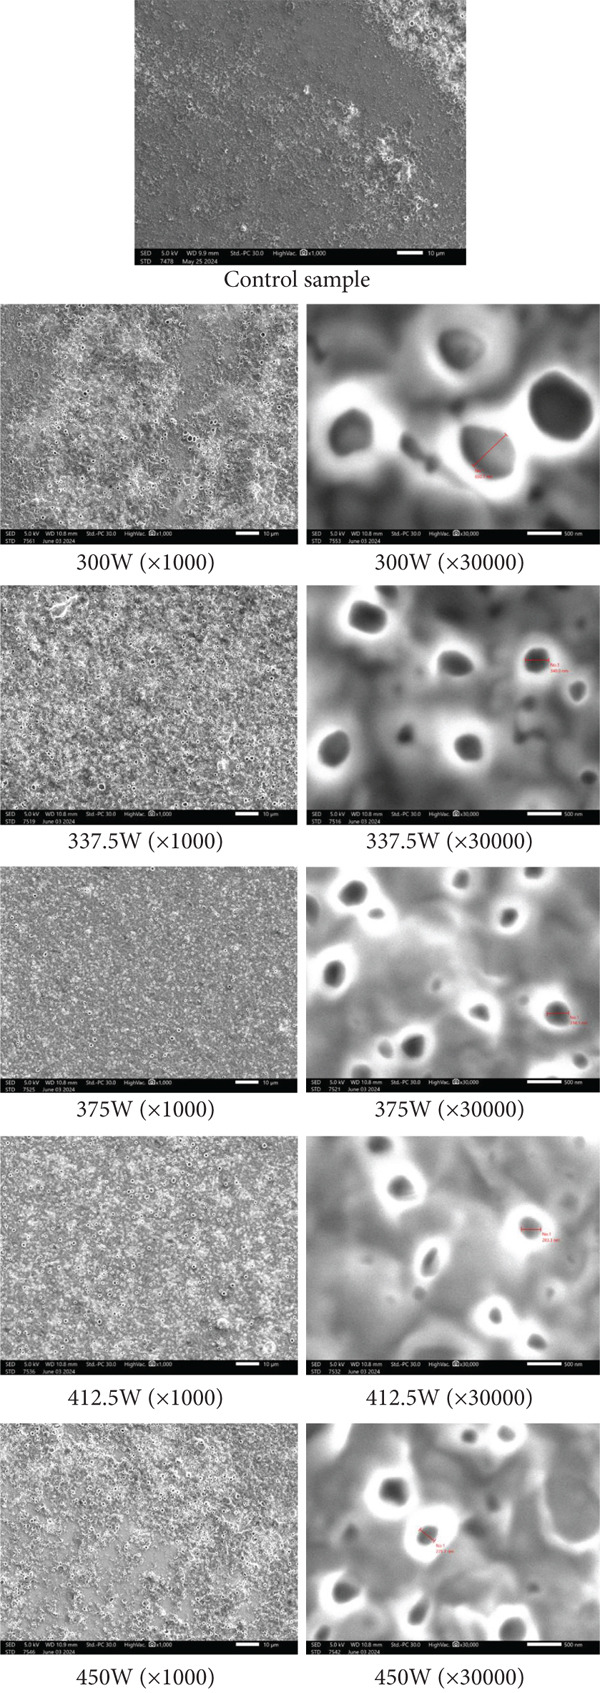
Effect of ultrasonic power on fat globule size of banana dragon fruit ice cream product.
